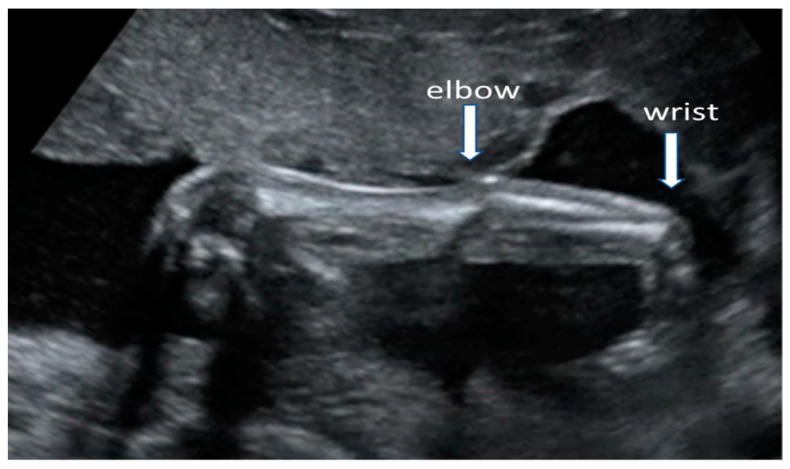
Ultrasound scan of a 22 weeks fetus with arthrogryposis multiplex congenita showing extended right upper extremity with fixed joints: elbow in extensions and wrist in flexion.
